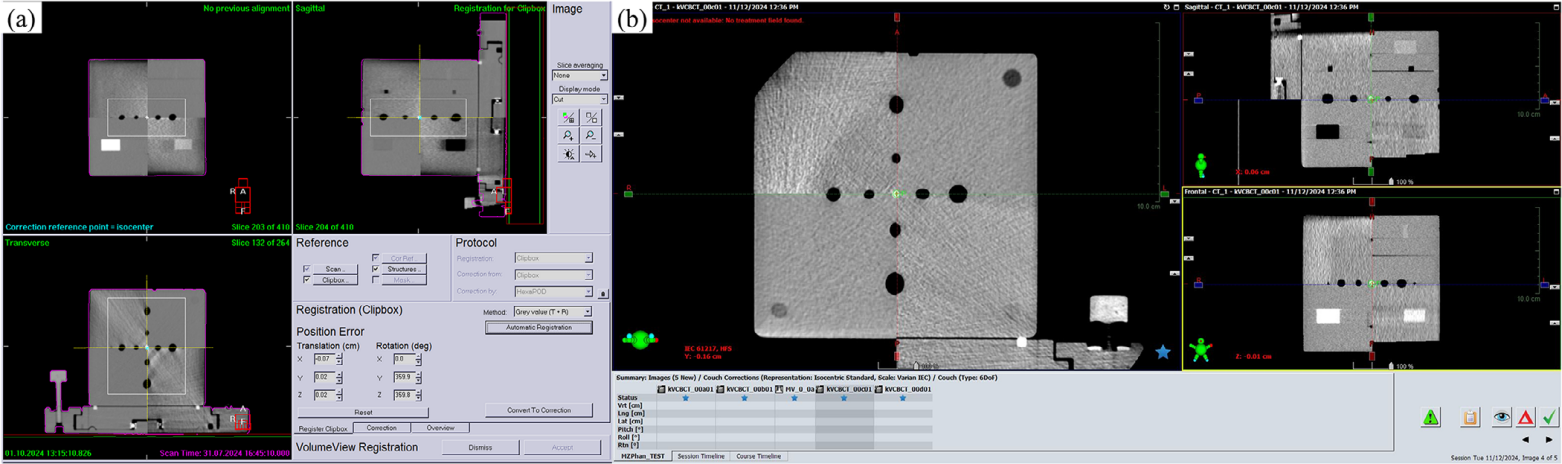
Image registration for coincidence check using (a) XVI CBCT and (b) OBI CBCT.
